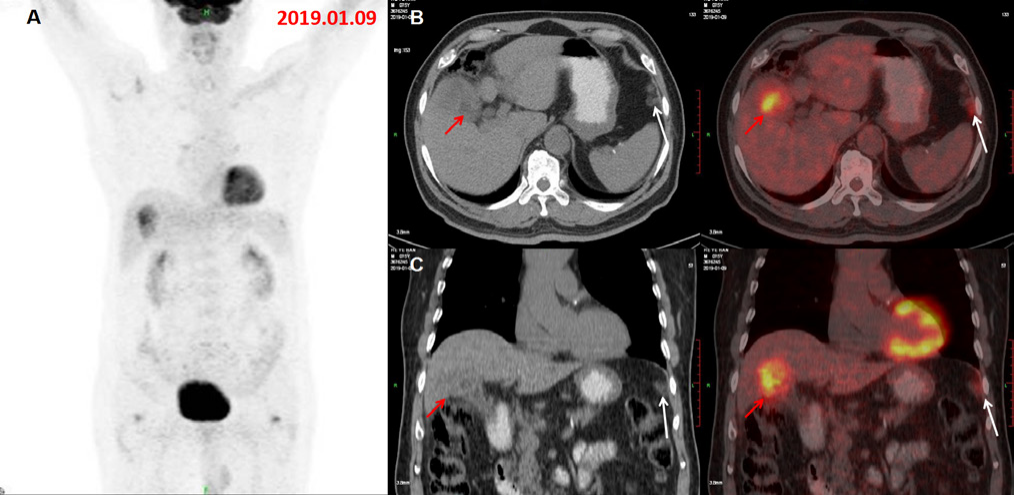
The MIP of the concurrent ^18^F-FDG PET/CT (A) demonstrated two foci
